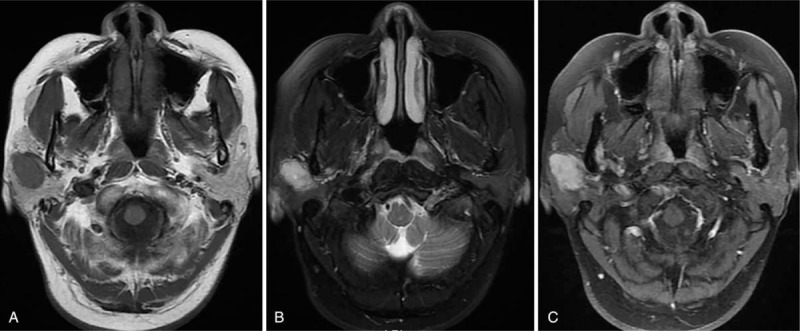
(A and B) The lesion exhibited a solid iso-intensity nodule on T1-weighted images, and high signal intensity with central spot higher signal intensity on T2-weighted fat-suppression images; (C) Axial enhanced images revealing lesion with obvious enhancement and mottled non-enhancement area.
